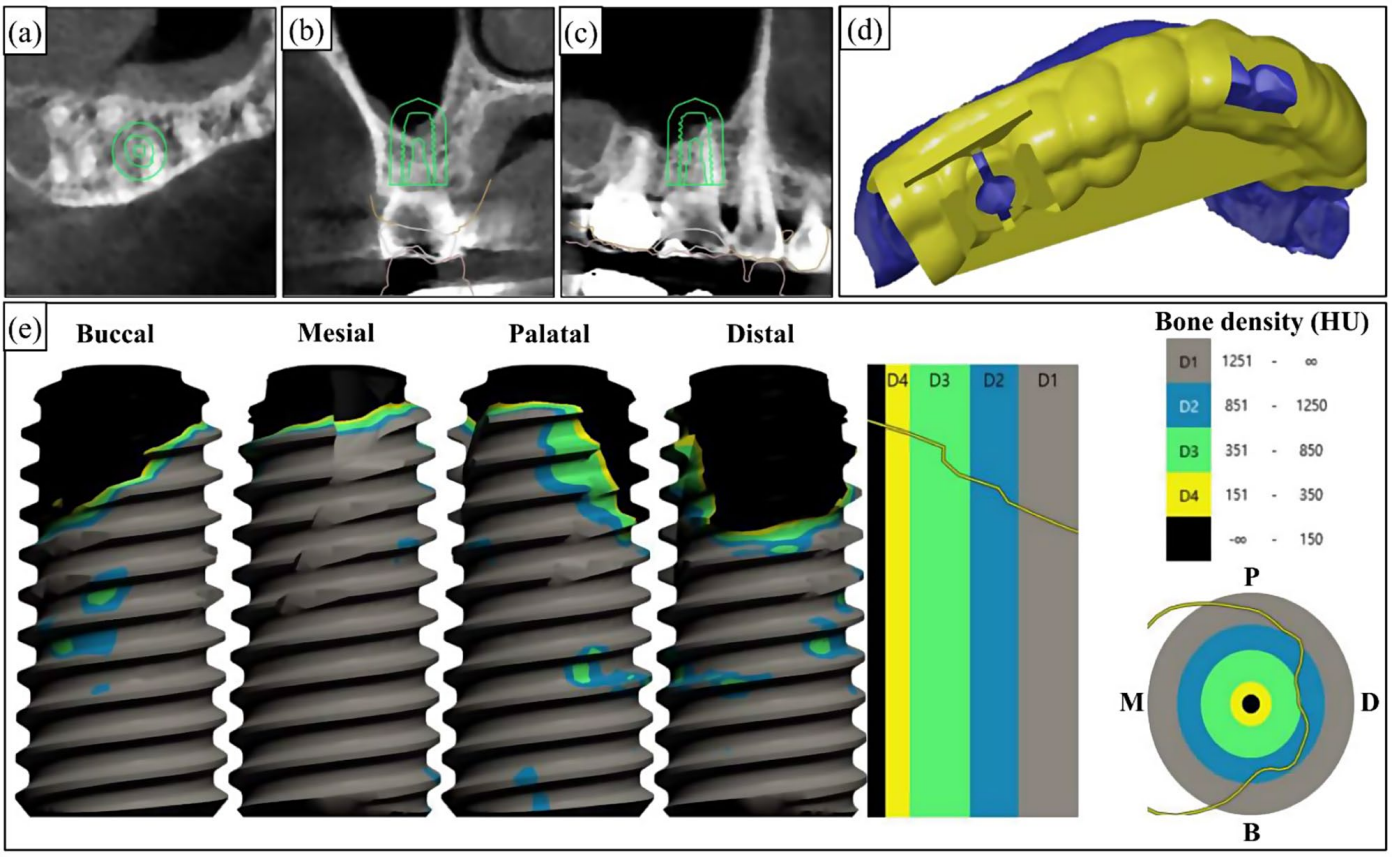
Flow of the digital surgical guide protocol. (a-c) Preoperative cone-beam computed tomography (CBCT) images and virtual implant position planning. (d) Virtual design and fabrication of the surgical guide. (e) Predicted bone-to-implant contact area according to bone density (HU; Hounsfield unit, D1; ≥1251 [gray], D2; 1250 − 851 [blue], D3; 351–850, [green] D4; 151–350, [yellow] None; ≤ 150 [black])
